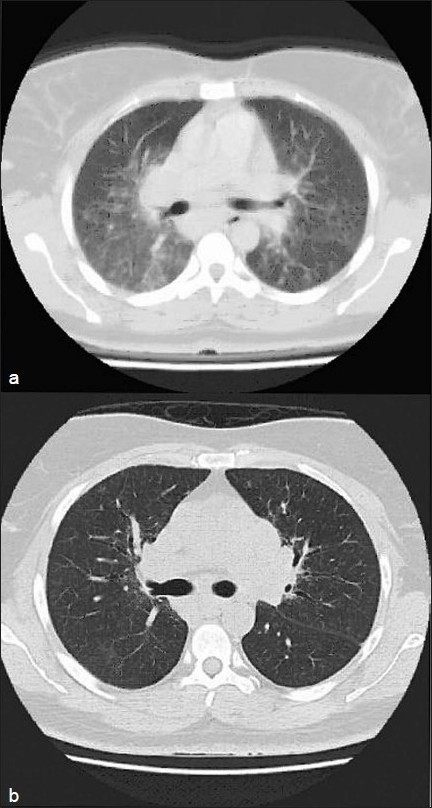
Patient 2: (a) HRCT scan showing widespread nodules with patchy areas of ground glass attenuation. (b) HRCT scan obtained at the 5-year follow-up showing regression of ground glass attenuation with treatment. Clinical characteristics of the patient are shown in Table 4
